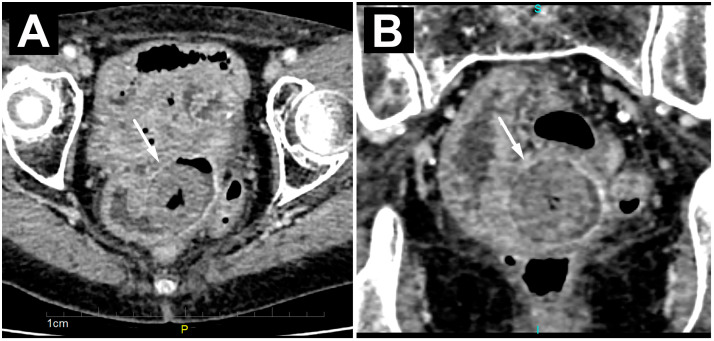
Axial (A) and coronal (B) CT images of an enclaved bezoar (white arrow) level with a ring-like, circumferential thickening of the ileum wall, with consequent proximal dilatation of the small bowel loops.
